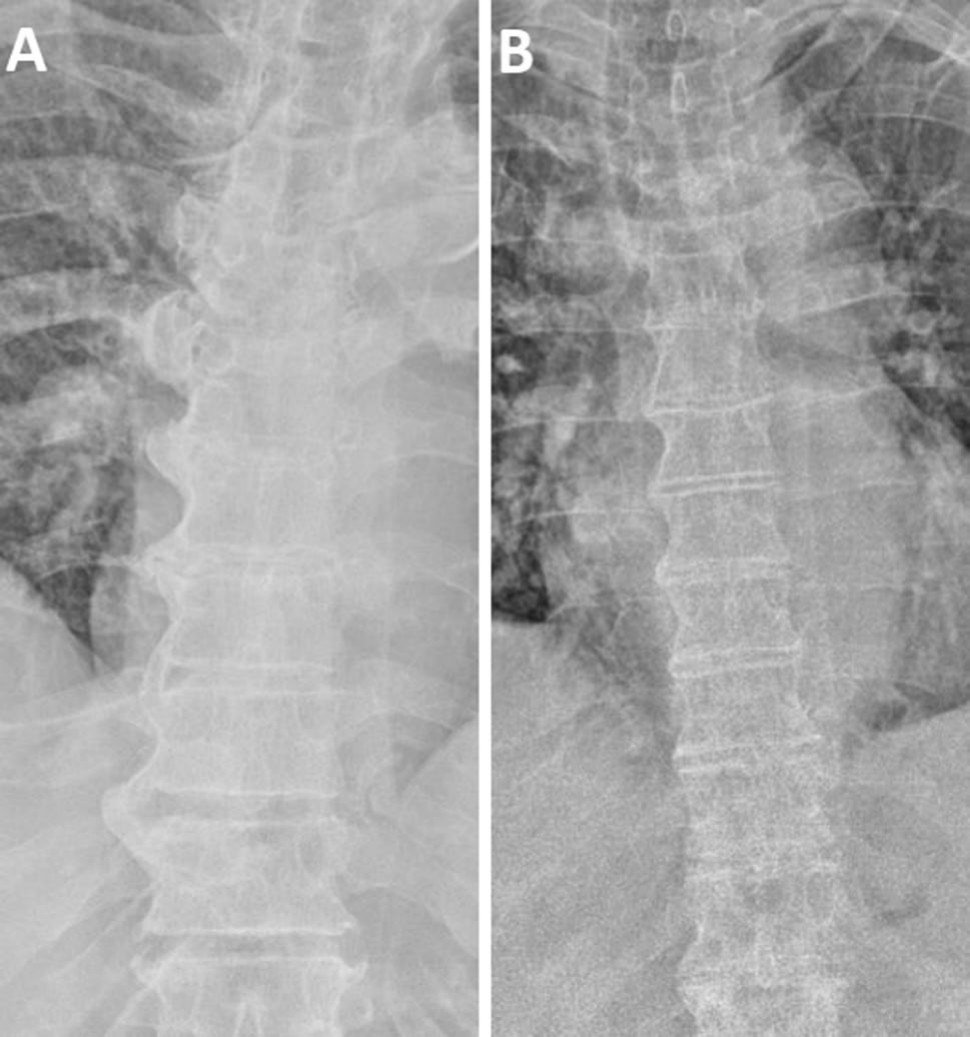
Coarse and smoother ossification patterns in DISH. a Anterior–posterior radiograph showing coarse flowing ossification of the lumbar spine with large ossification of the anterior lateral ligament; b anterior–posterior radiograph showing bone bridges with smoother ossification pattern
